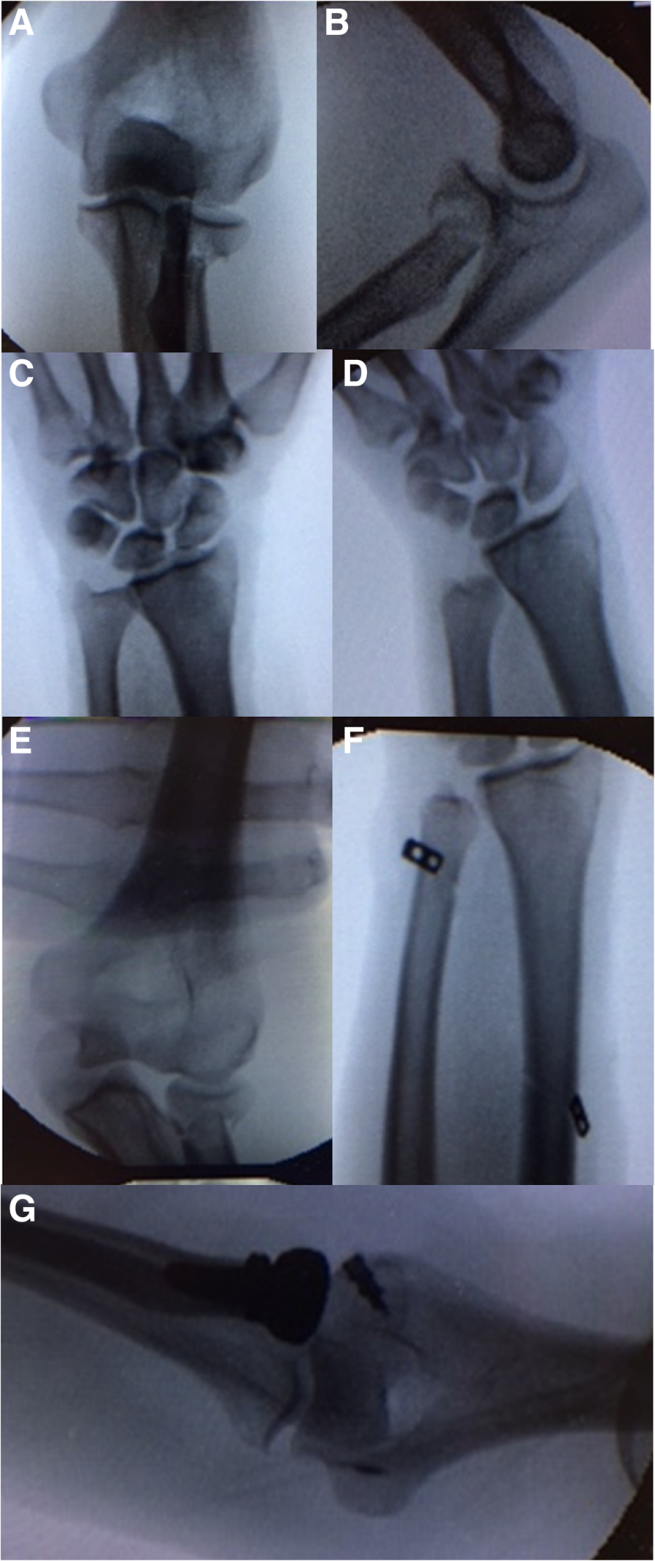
Clinical case 2: Acute Undetected at Imminent Evolution ELI pattern. Pre-operative X-ray of case n. 2: it is evident the radial head fracture without evident signs of high energy trauma (a, b). The DRUJ seemed aligned ad regular X-Ray (c). Performing the stress test under C-arm view the forearm longitudinal instability was detected (d, e). The treatment consisted in radial head prosthesis positioning (g), IOM plasty and collateral ligaments reconstruction (f)
